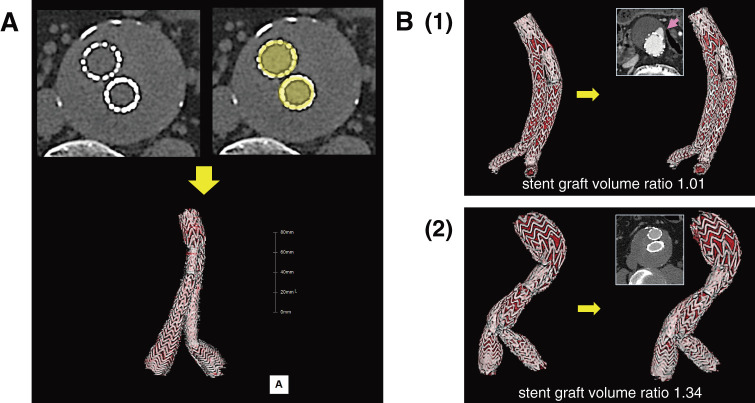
Stent graft volume measurement. (A) Axial plain CT images before and after tracing the stent border lines, and a 3-dimensional image reconstructed from plain CT images. Stent graft volume is calculated as the integrated value by tracing the outer stent border from top to bottom in the axial images of plain CT. The inner area of the stent is displayed in red if a 3-dimensional image is reconstructed from plain CT images, and the red area in the 3-dimensional image is not contrasted. (B) Images of 2 cases as examples: (1) the stent graft with Type II endoleaks (T2EL) *(arrow)* and (2) the stent graft without T2EL. The 3-dimensional image of the stent graft within 1 month after EVAR (left) and the axial CT and 3-dimensional images of the stent grafts 2 years after EVAR (right) are shown for each case. The axial contrast-enhanced CT images clarify the existence or absence of T2EL, but stent graft volume is measured on plain CT images. The SgVs were 54.5 mL initially and 55.0 mL 2 years after EVAR, and the SgV ratio was calculated as 1.01 in Case 1. The SgVs were 83.8 mL initially and 111.9 mL 2 years after EVAR, and the SgV ratio was calculated as 1.34 in Case 2. EVAR: endovascular aneurysm repair; ePTFE: expanded polytetrafluoroethylene; CT: computed tomography; SgVs: stent graft volumes
